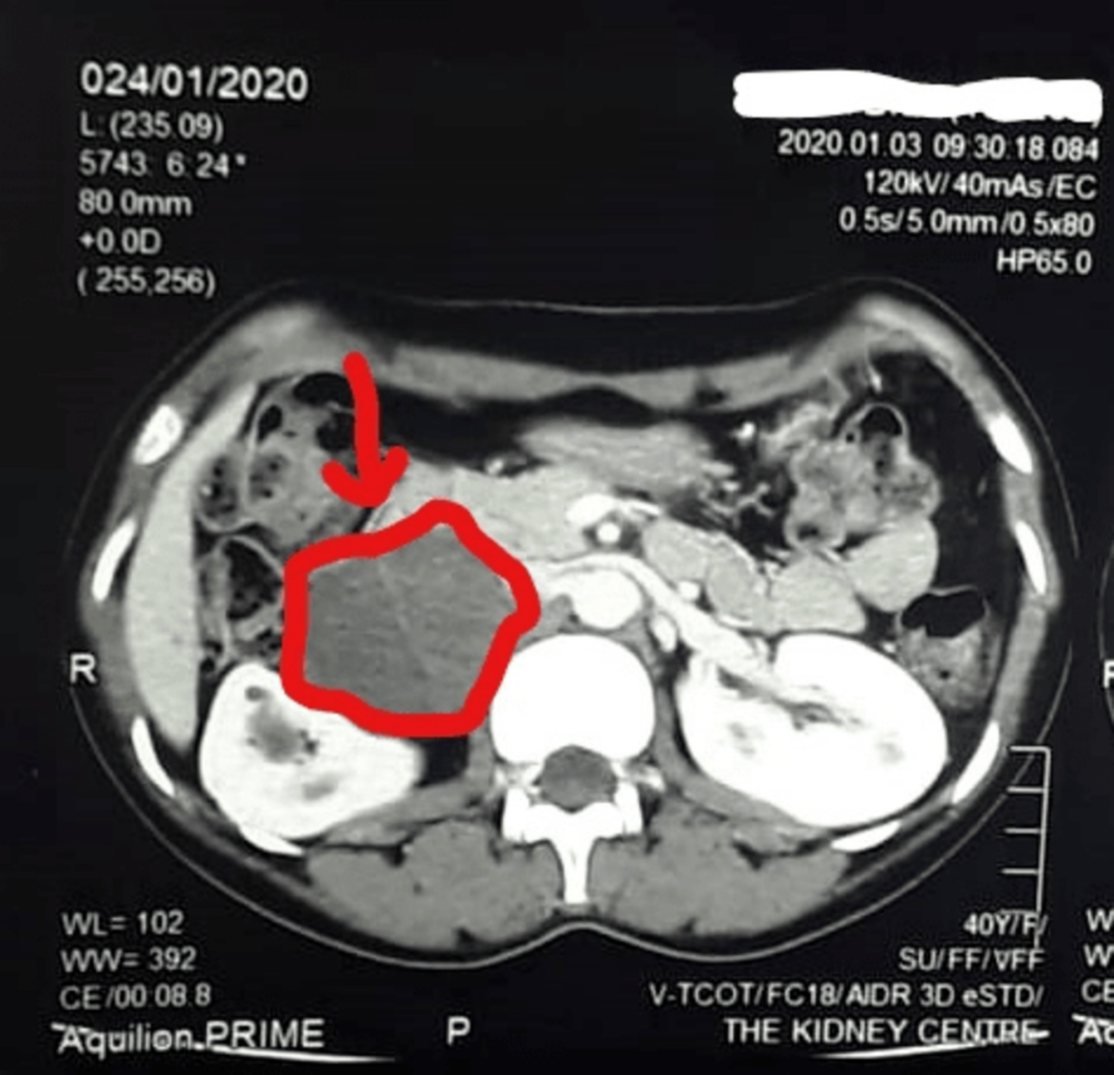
CT scan (axial view) of the abdomen showing a right-sided mass.
